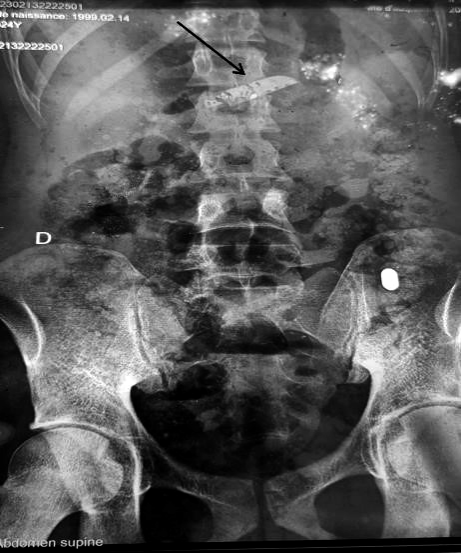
Radiographie de l'abdomen sans préparation montrant une image radioopaque de couteau
